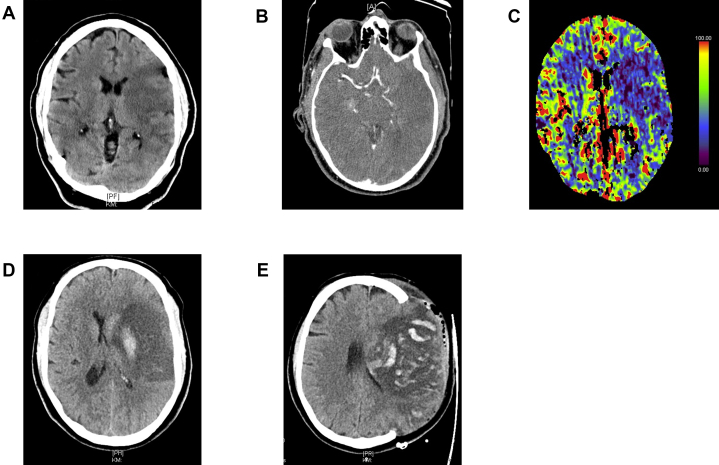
Computed Tomography Showing a Large Infarction of the Left Middle Cerebral Artery (A) Baseline with early infarct demarcation. (B) Angiography showing occlusion in the M1 segment. (C) Perfusion mismatch. (D) Follow-up 1 day later showing progressive cerebral edema. (E) After decompressive hemicraniotomy with secondary bleeding in the infarction.
